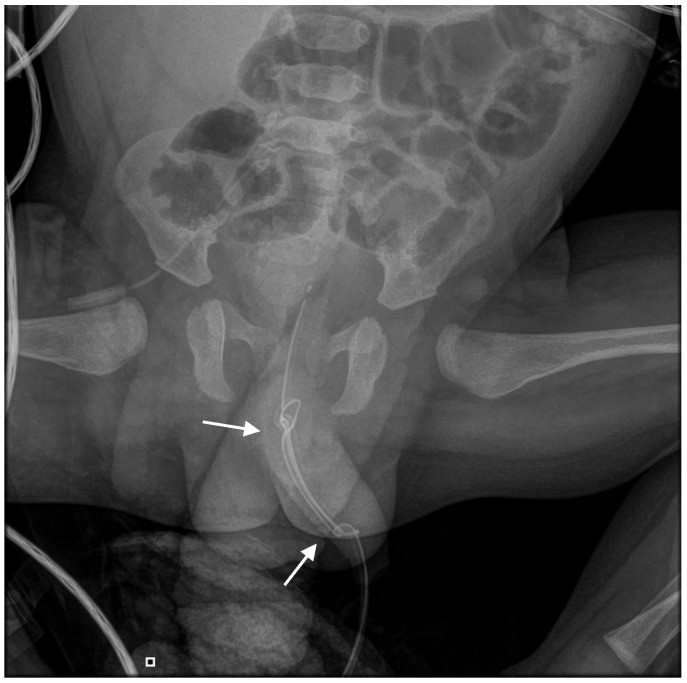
X-ray of the lower abdomen of the patient, showing the double knot located at the distal urethra and the single knot located at the proximal urethra (near the bladder neck) of the urinary catheter (the arrows are pointing at the knots).
